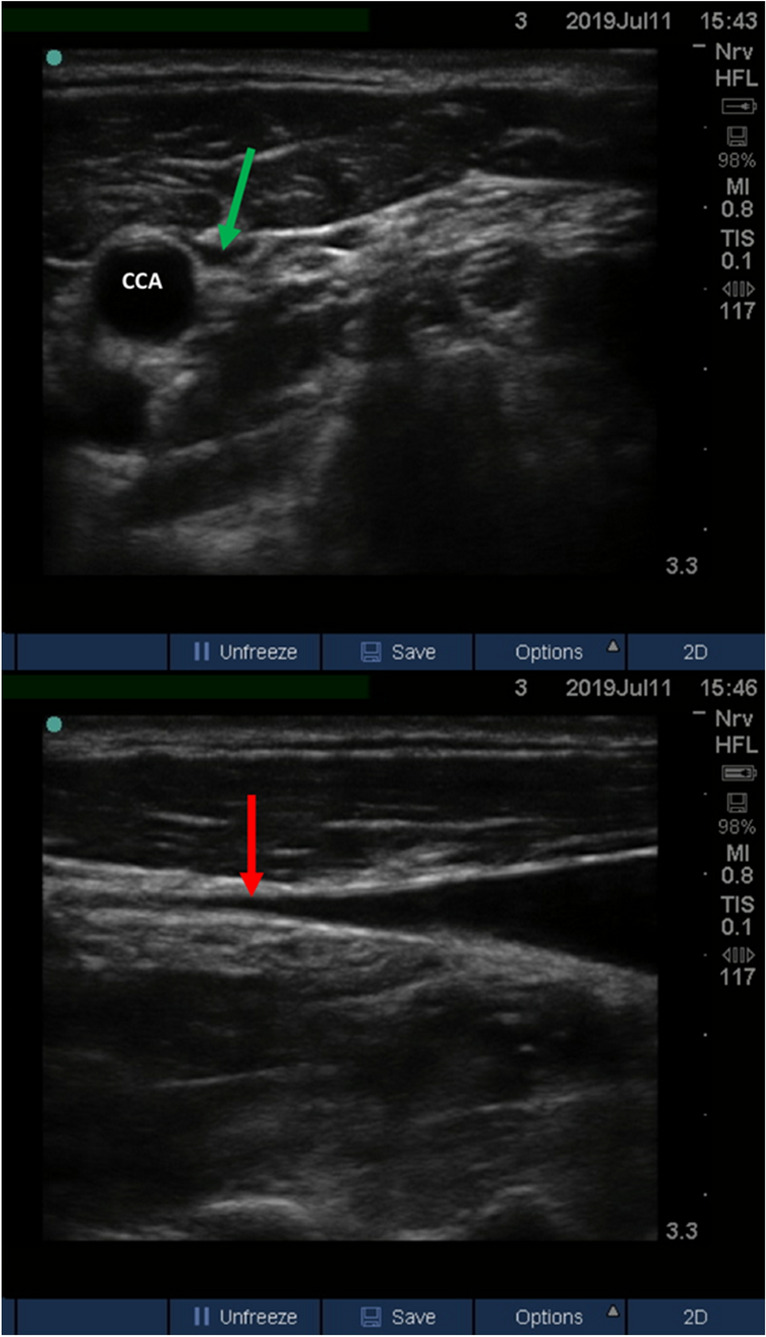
Ultrasound-measured jugular venous pressure imaged in a semi-recumbent position. Top: transverse view of a collapsed IJV. The green arrow points towards the collapsed IJV. Bottom: longitudinal axis of the IJV. The IJV tapers until reaching the point of collapse. The red arrow indicates the point at which the vein is collapsed. *CCA* common carotid artery, *IJV* internal jugular vein
